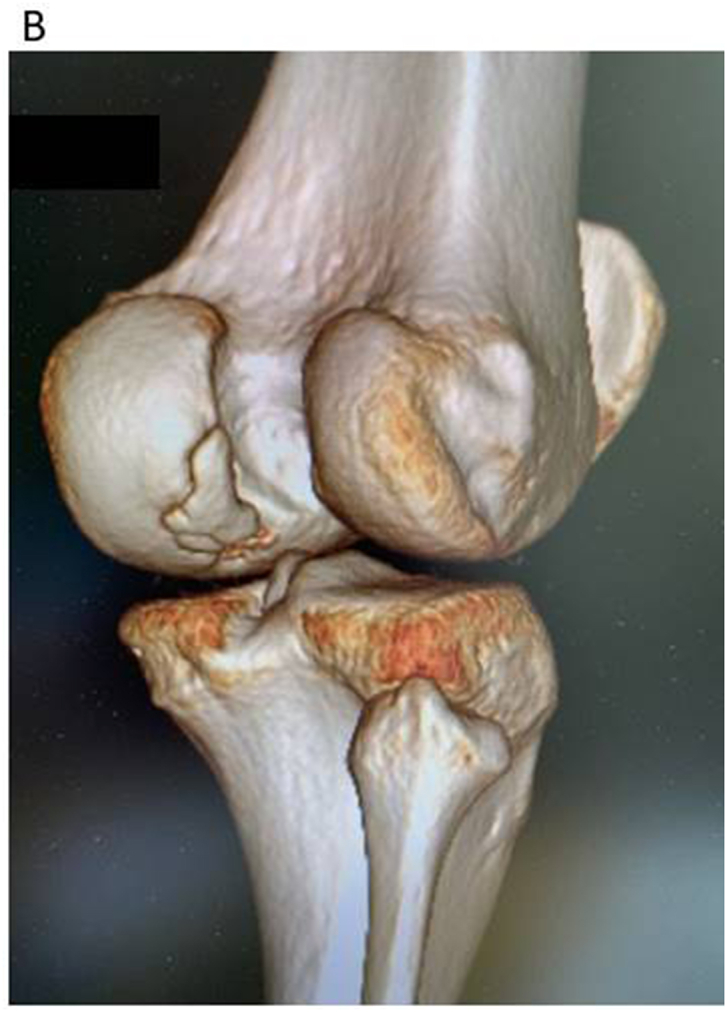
The same lesion as on Fig. 1A but now seen from a view possible to get with a 3D CT scan reconstruction.The exact size could be calculated from such a reconstruction and also the reachability.
